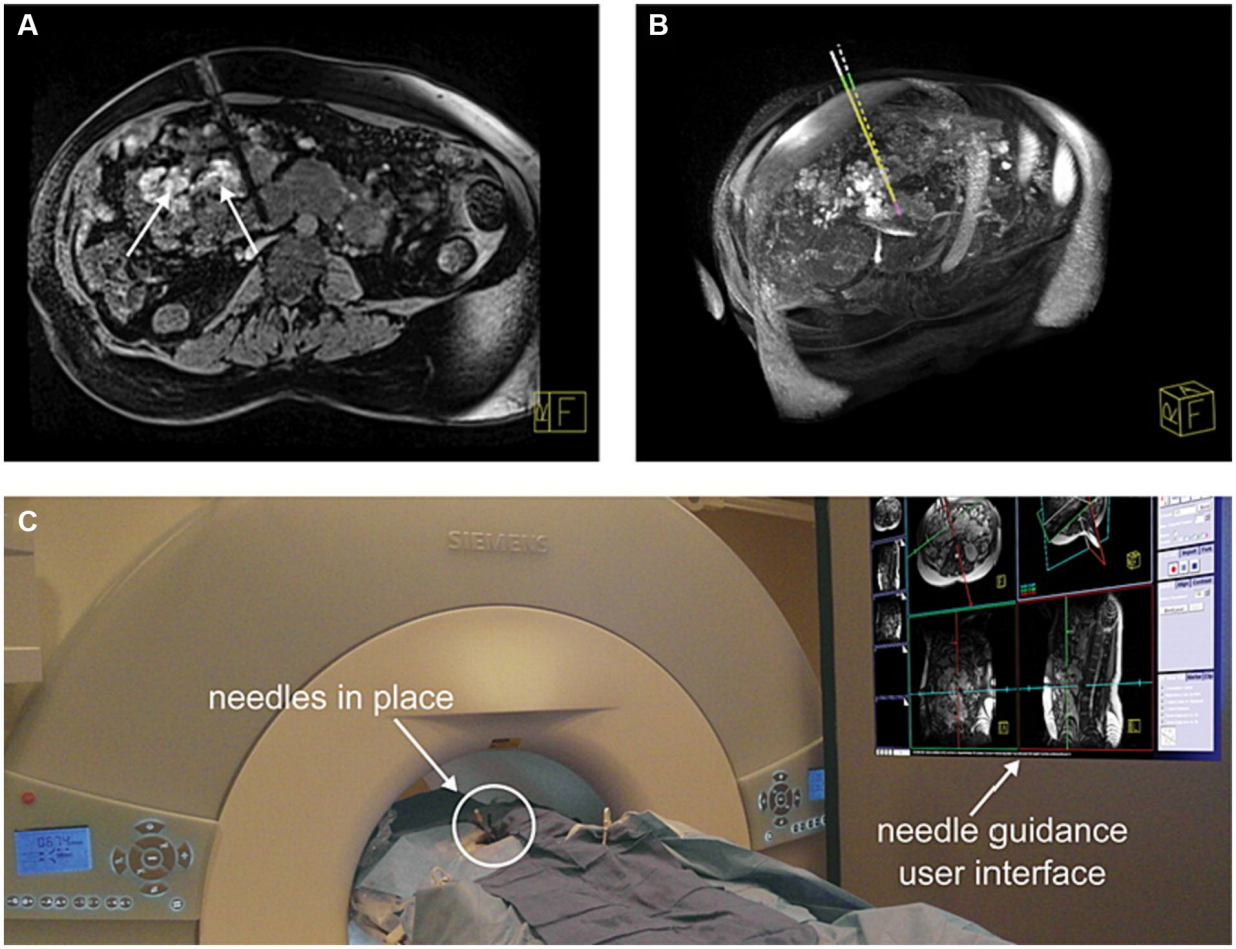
Successful needle placements (four needles) for sclerotherapy of a complex intraperitoneal venous malformation (VM) in a 40-year-old adult with Klippel–Trenaunay syndrome who could not be treated by ultrasound and x-ray fluoroscopy-guided treatment. (A) Verification dataset of needle placement into a VM adjacent to vena cava and other critical structures. Areas of the VM treated earlier in this procedure with gadolinium DTPA-doped SDS 3% astill show enhancement in the image (arrows). (B) Comparison between planned (dashed line) and actual needle trajectory. (C) Patient in the scanner with needle guidance user interface displayed on a screen in the MR scanner room. Reproduced with permission, John Wiley and Sons, Inc. (40).
